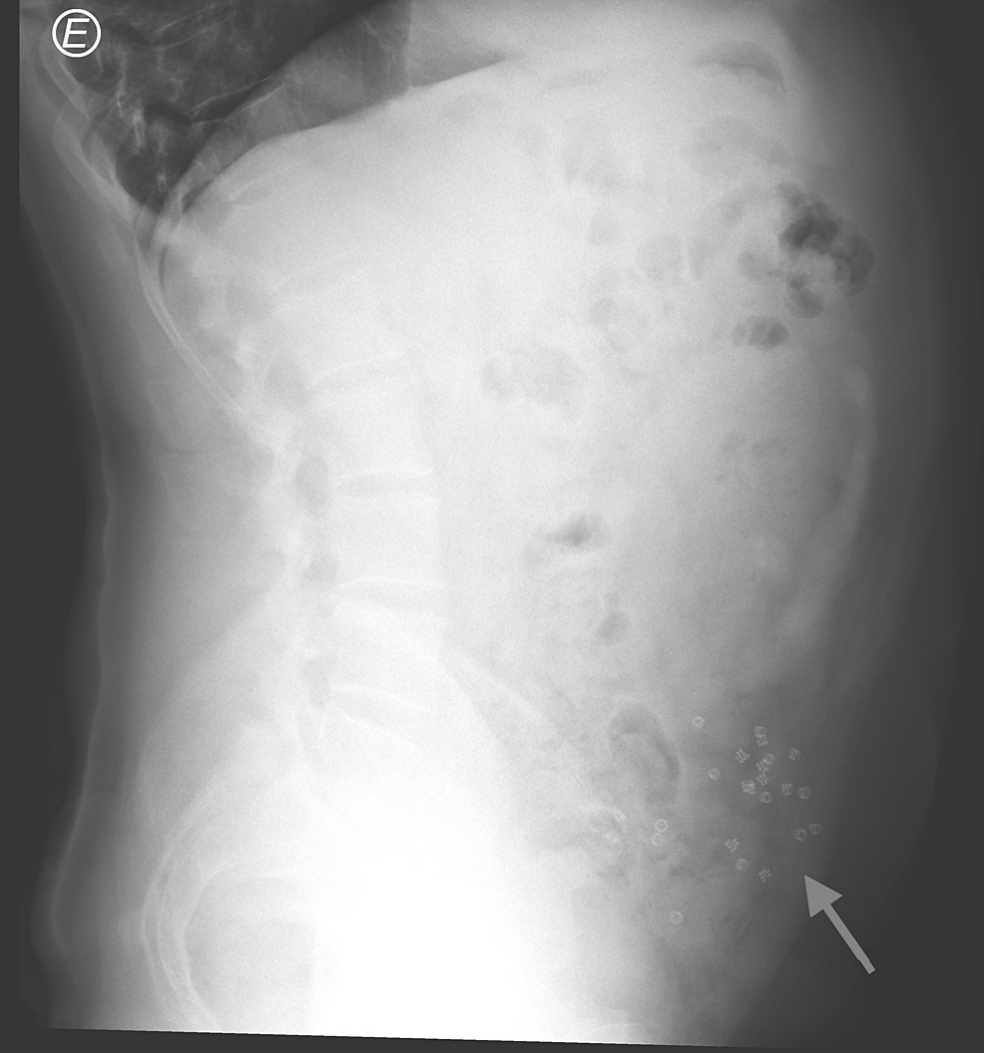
Abdominal X-ray showing multiple tacks (arrow), with an absence of free air or signs of ileus
